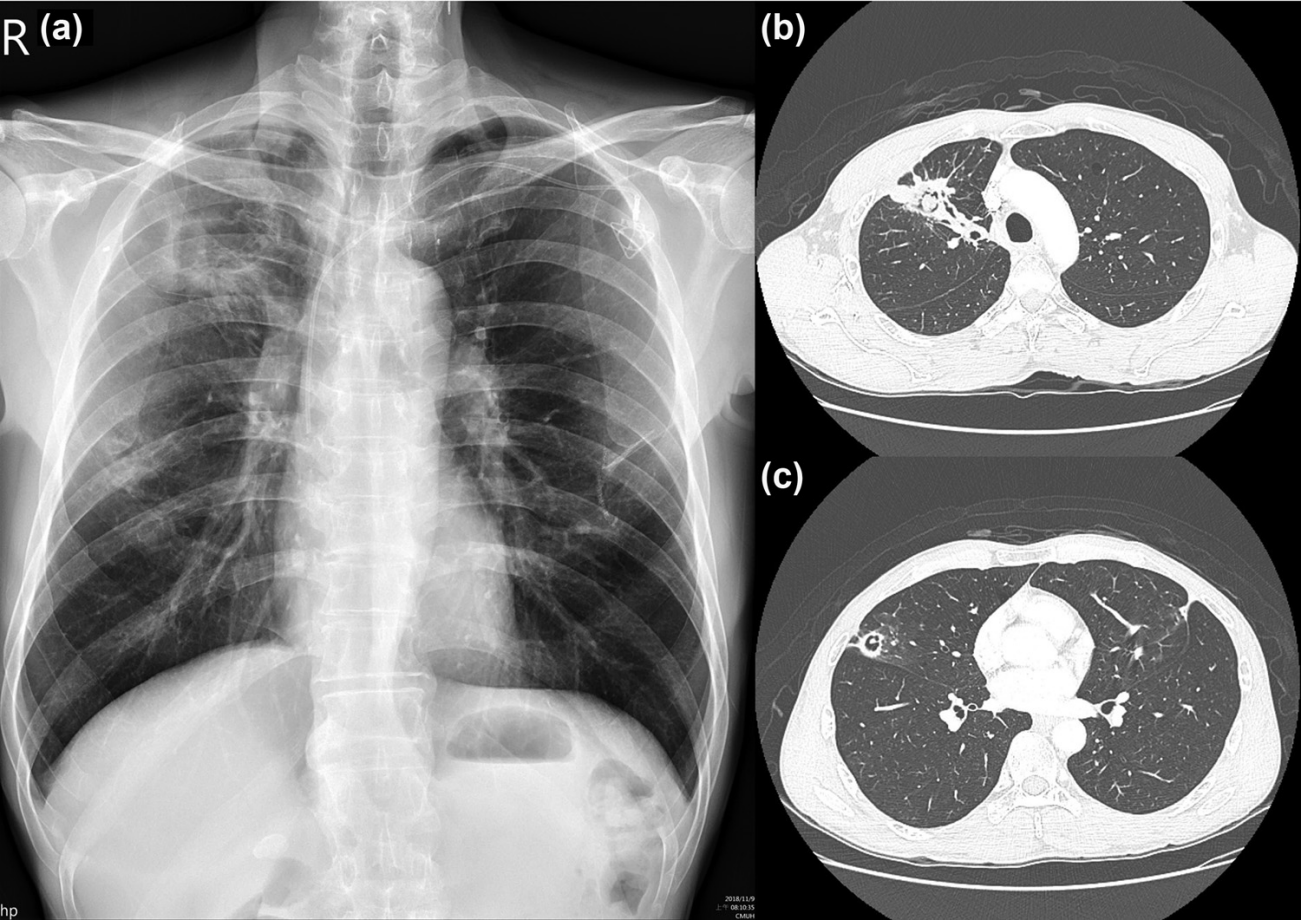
(a–c) Chest radiography and computed tomography following voriconazole administration and subsequent chemotherapy: regression of the pulmonary lesions on follow-up.
